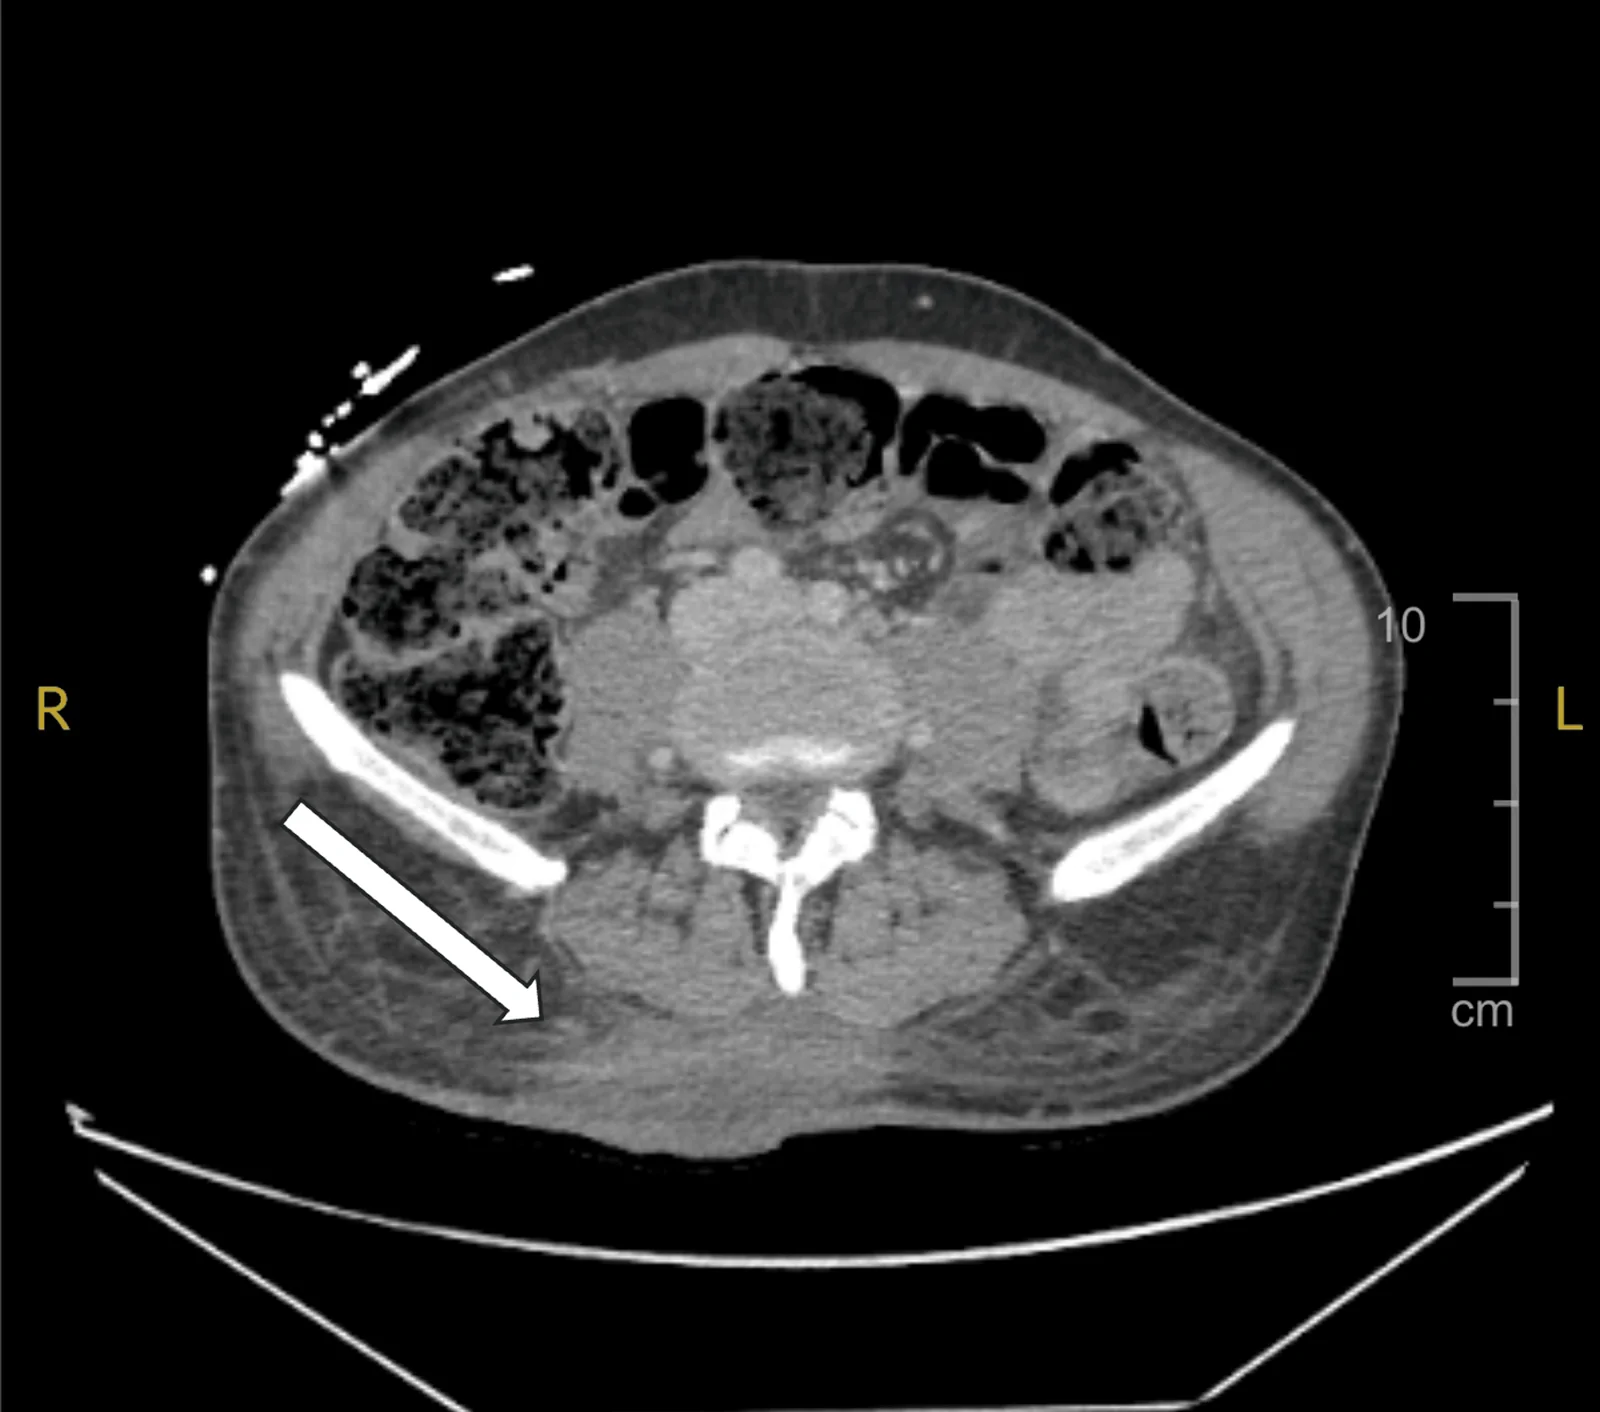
Computed tomography (CT) axial view of the abdomen and pelvis The image displays subcutaneous 5.8 x 4.6 x 10.3 cm multiloculated abscess in the right mid-to-lower lumbar subcutaneous tissue with surrounding fat stranding and skin thickening, poorly delineated from adjacent muscle fascia and without connection to the skin surface (arrow).
